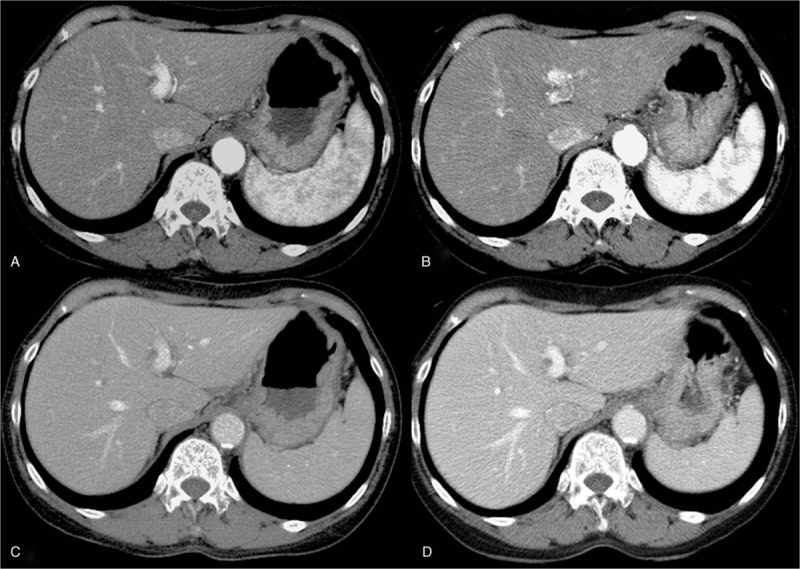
The patient was a 67-year-old woman with liver cirrhosis and her body height and weight were 164 cm and 54 kg. She happened to be included in both CT protocols. Axial images of (A) 120 kVp – hepatic arterial phase, (B) 100 kVp – hepatic arterial phase, (C) 120 kVp – portal venous phase, and (D) 100 kVp – portal venous phase are shown.
